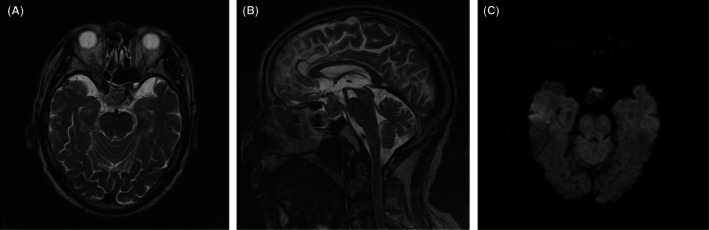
Magnetic resonance imaging of brain. Non‐contrast T2‐weighted axial section (A) coronal section (B), and diffusion‐weighted imaging axial section (C) of pituitary MRI at presentation demonstrating right shifted sellar tumor enlargement followed by hemorrhagic infarction. It is compatible with pituitary apoplexy.
